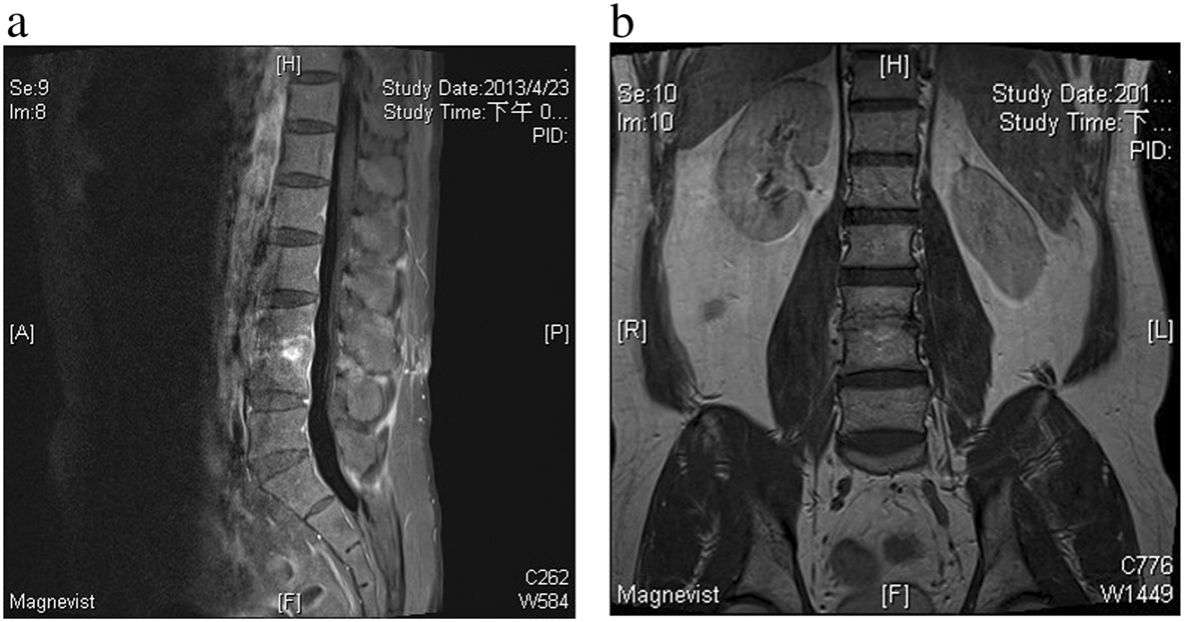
Magnetic resonance imaging showed significant shrinkage of the vertebral osteomyelitis, discitis, and paraspinal abscesses. (a and b) Magnetic resonance imaging (MRI) showed significant shrinkage of the vertebral osteomyelitis, discitis, and paraspinal abscesses, although there were still abnormal signals and enhanced lesions in the disc adjacent to the affected vertebral bodies.
